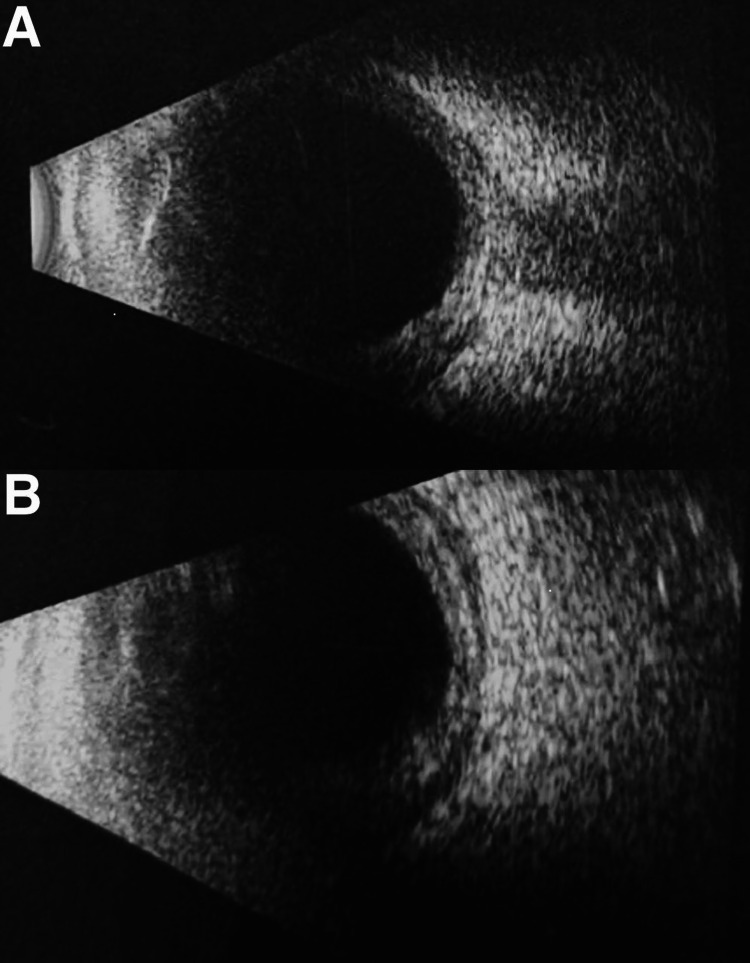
B-scan ultrasonography: (A) classic T-sign; (B) thickened sclera with fluid collection in the sub-Tenon space.
